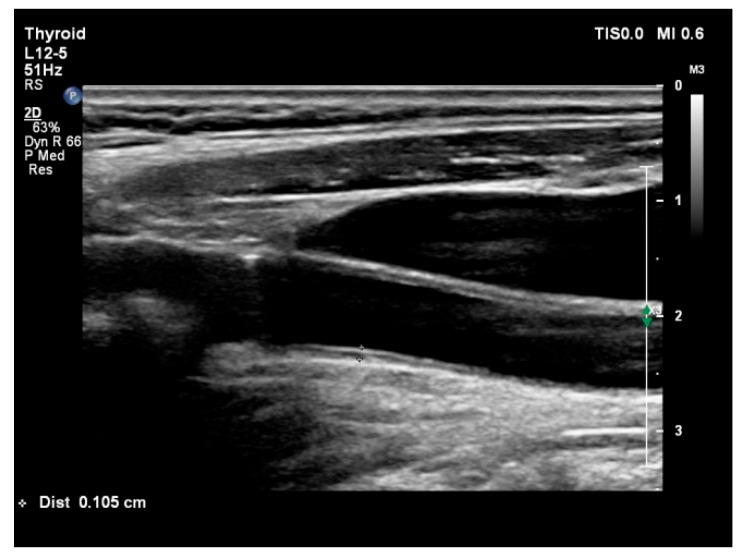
Increased common carotid intima–media thickness Doppler ultrasound image in patients with NSTEMI.
